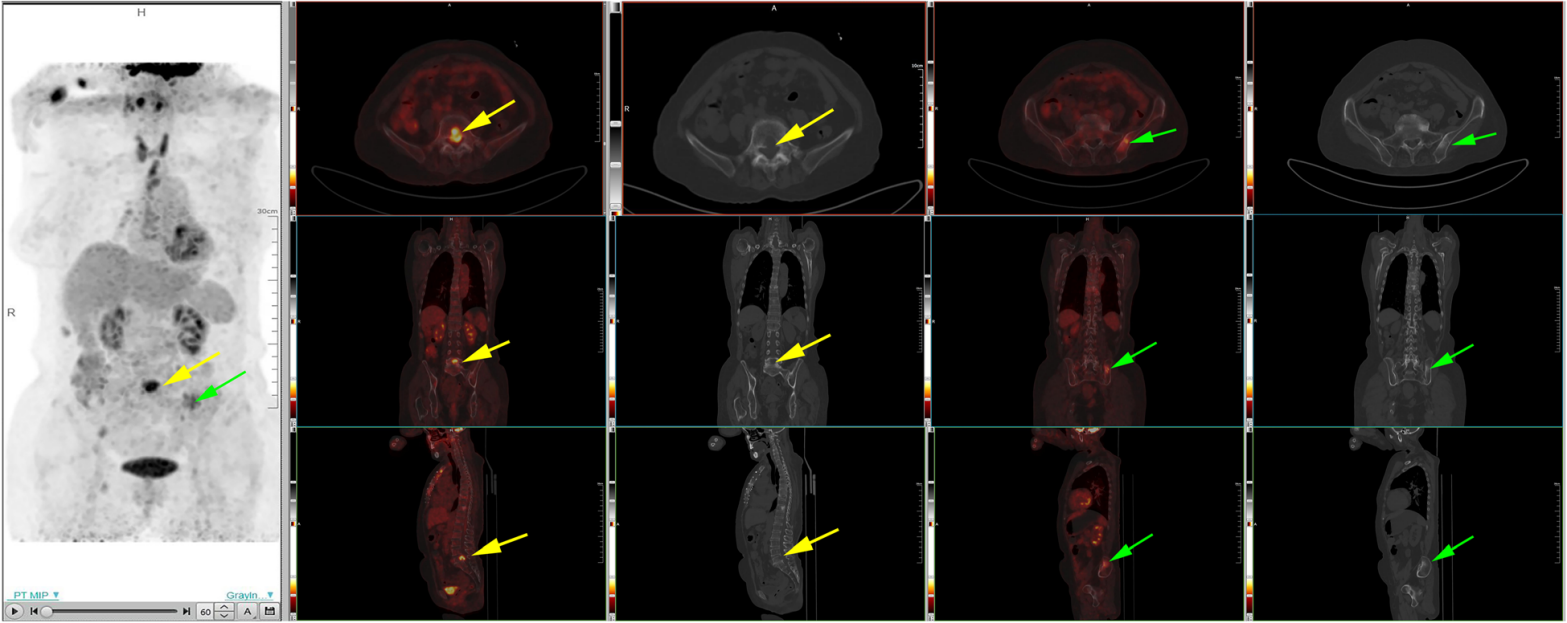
^18^F-FDG PET/CT showed multiple hypermetabolic lesions which was consistent with lytic bone changes in the T5, T10, and L5 vertebra, as well as bilateral ilium and right ischium
